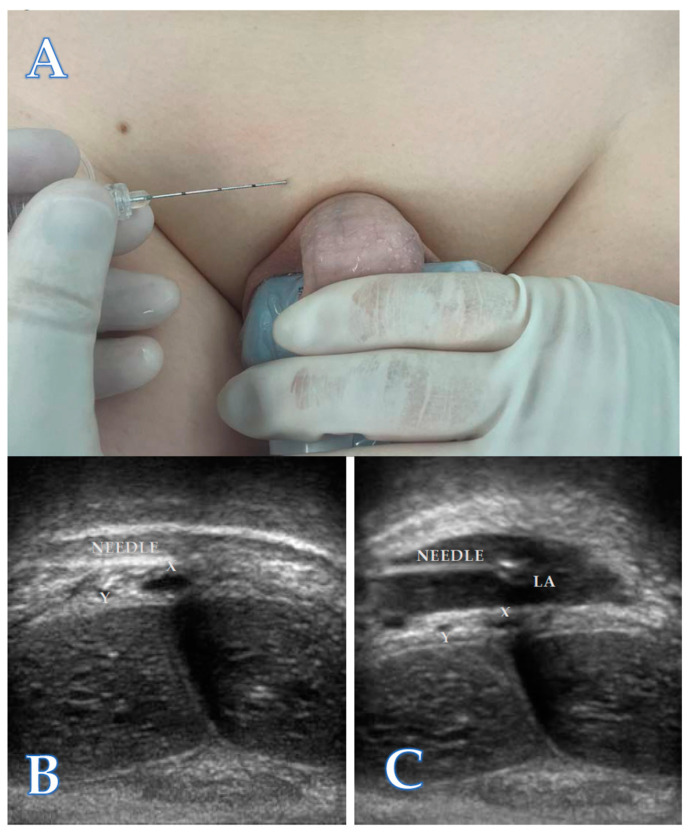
Probe positioning (A) and echographic visualization of the ultrasound-guided dorsal penile nerve block before (B) and after (C) LA injection. Dorsal penile arteries (Y), deep dorsal penile vein (X).
